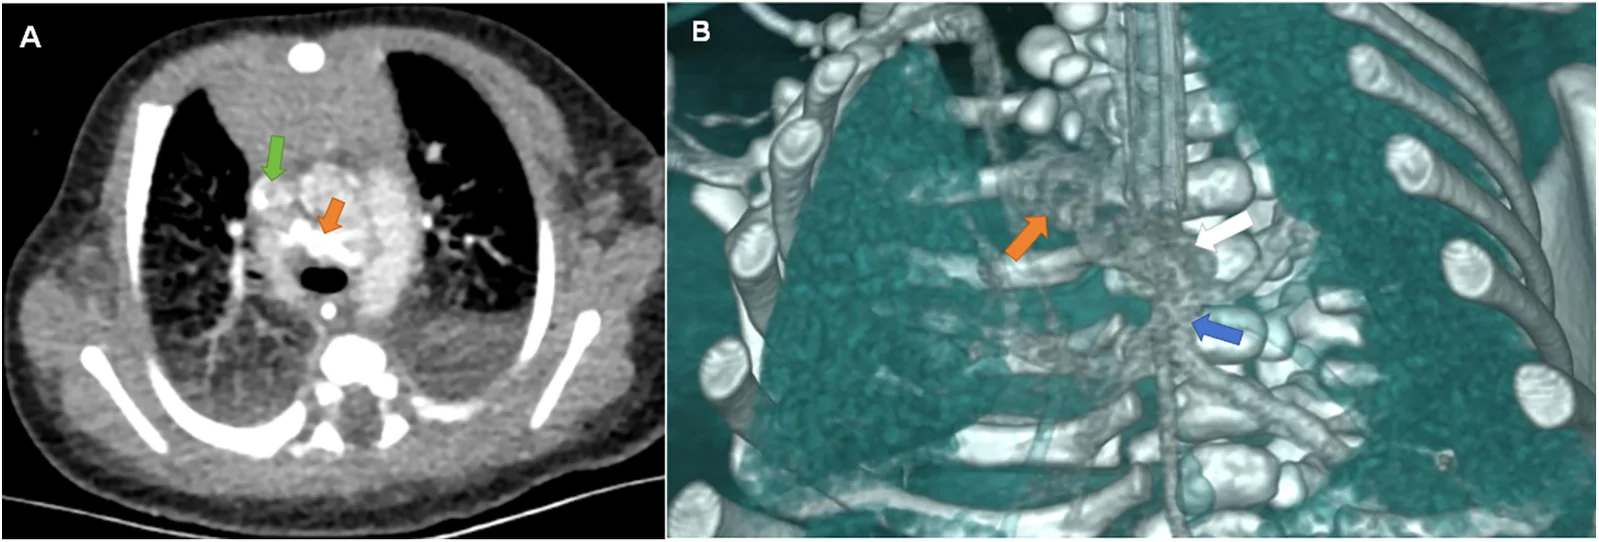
Cardiac CTA (A) with three-dimensional reconstruction (B) demonstrates a CPVC (blue arrow) and ascending vertical vein (white arrow), forming a tortuous vascular mass (orange arrow) anterior to the trachea and posterior to the SVC (green arrow) in the patient. *CTA*, computed tomographic angiography; *CPVC*, common pulmonary venous chamber; *SVC*, superior vena cava.
